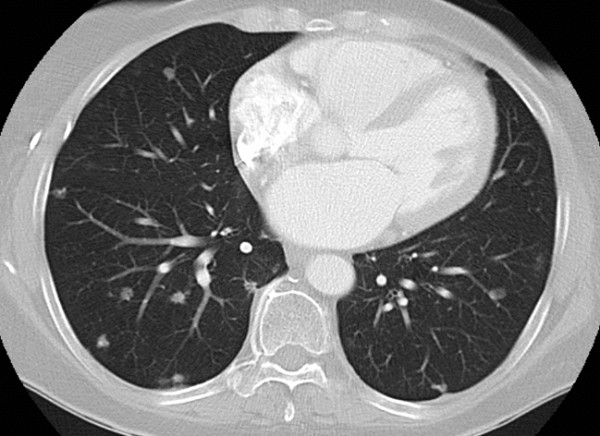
Computed tomography scan of the thorax performed after seven years of treatment showing the lack of any significant disease progression during thalidomide therapy.
